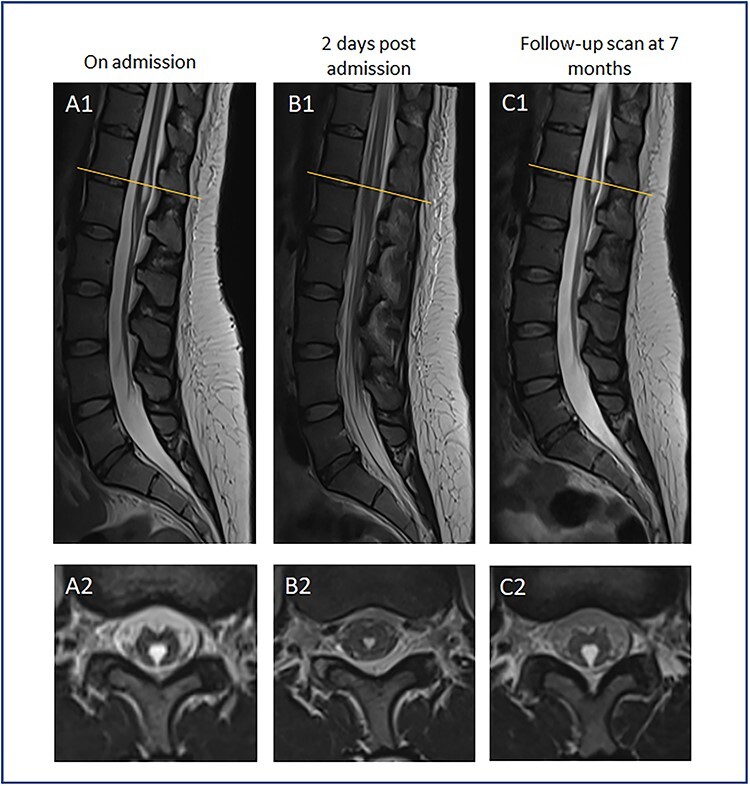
MR spinal imaging during admission and at follow-up. T2 weighted MR sagittal (A1-C1) and axial (A2-C2) images at T12/L1 level (orange line). A—Admission MRI, B—MRI 2 days post admission, C—7 month follow-up scan. A1 + 2 at initial presentation with expanded TV cyst compressing conus causing acute neurological deficit. B1 + 2 showing conus appearances post TV cyst rupture corresponding with resolution of neurological symptoms. C1 + 2 images at 7 month follow-up in keeping with cyst recollection with no corresponding neurological deficit.
